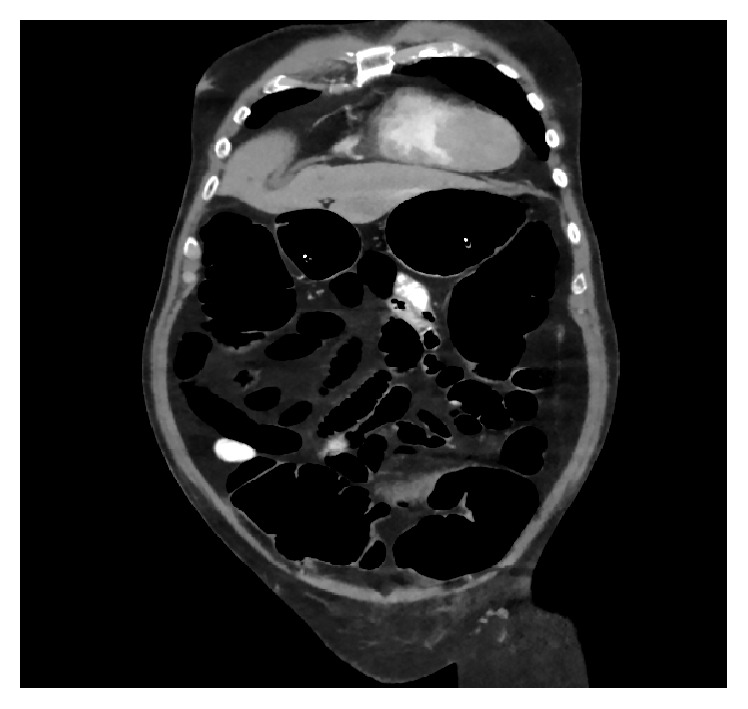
CT image of abdomen showing marked colonic dilation without any evidence of obstruction.
